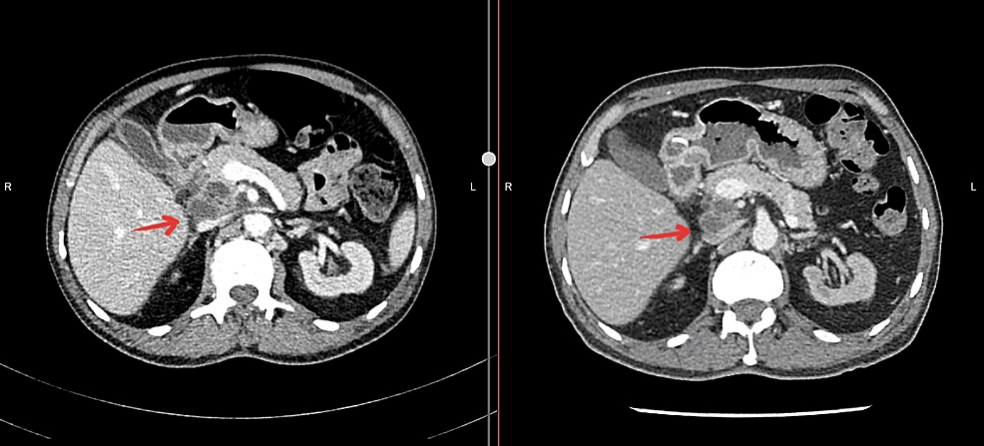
Comparison of CT abdomen performed before (Left) and after (Right) initiation of treatment. Significant reduction in the size of the central necrotic lymph nodes, three months after the initiation of antitubercular therapy.
